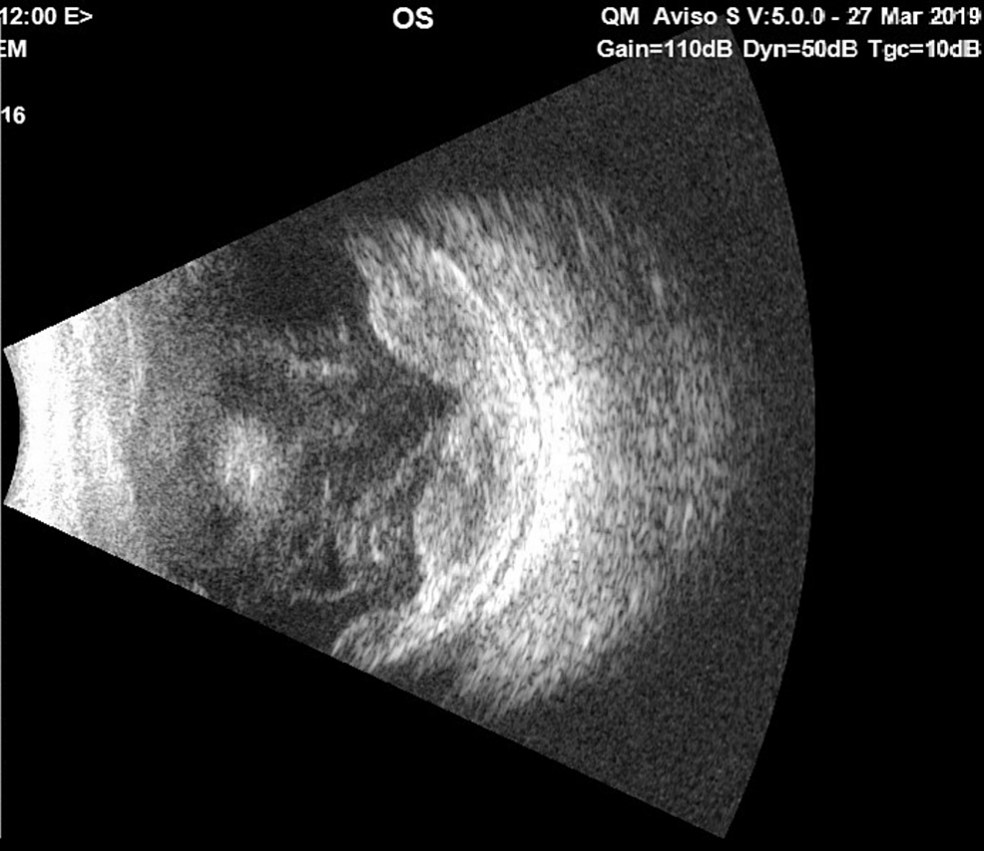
B-Scan ultrasonography of the eye at presentation showed choroidal detachment with subchoroidal opacity, vitreous strands, and scleral thickening.
